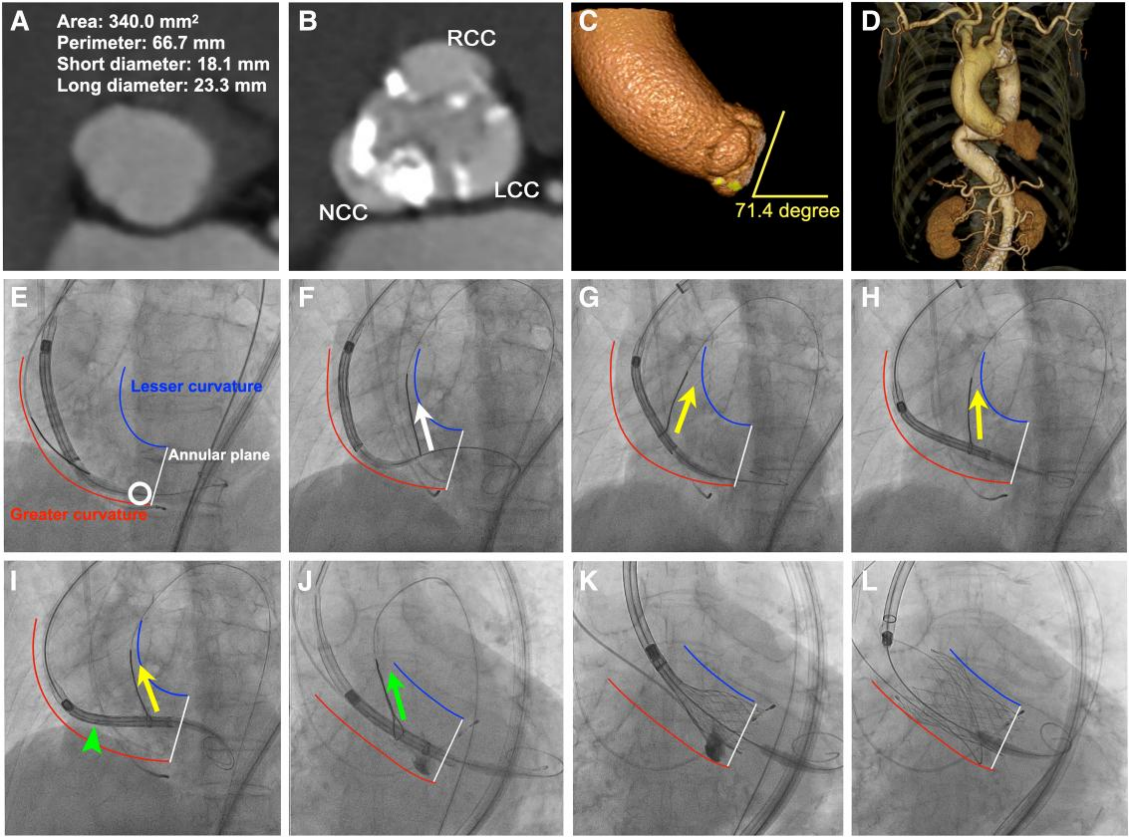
Snare-assisted delivery and coaxial optimization. (*A–D*) Pre-procedural computed tomography demonstrates severe calcification, particularly in the non-coronary cusp (NCC), along with an extremely horizontal ascending aorta (aortic root angle: 71.4°) and a tortuous descending aorta. (*E*) Bioprosthesis failed to cross the aortic valve because of extensive NCC calcification (white circle). (*F*) The nose cone was initially snared but failed to cross the aortic valve (white arrow). (*G–I*) Snare engagement at the lower third of the delivery capsule facilitated valve advancement (yellow arrows); however, non-coaxial alignment was observed (green arrowhead). (*J*, *K*) Snare engagement at the upper third of the capsule achieved proper coaxial alignment (green arrow). (*L*) Final result. White line: annular plane. Blue line: lesser curvature of the ascending aorta. Red line: greater curvature of the ascending aorta.
